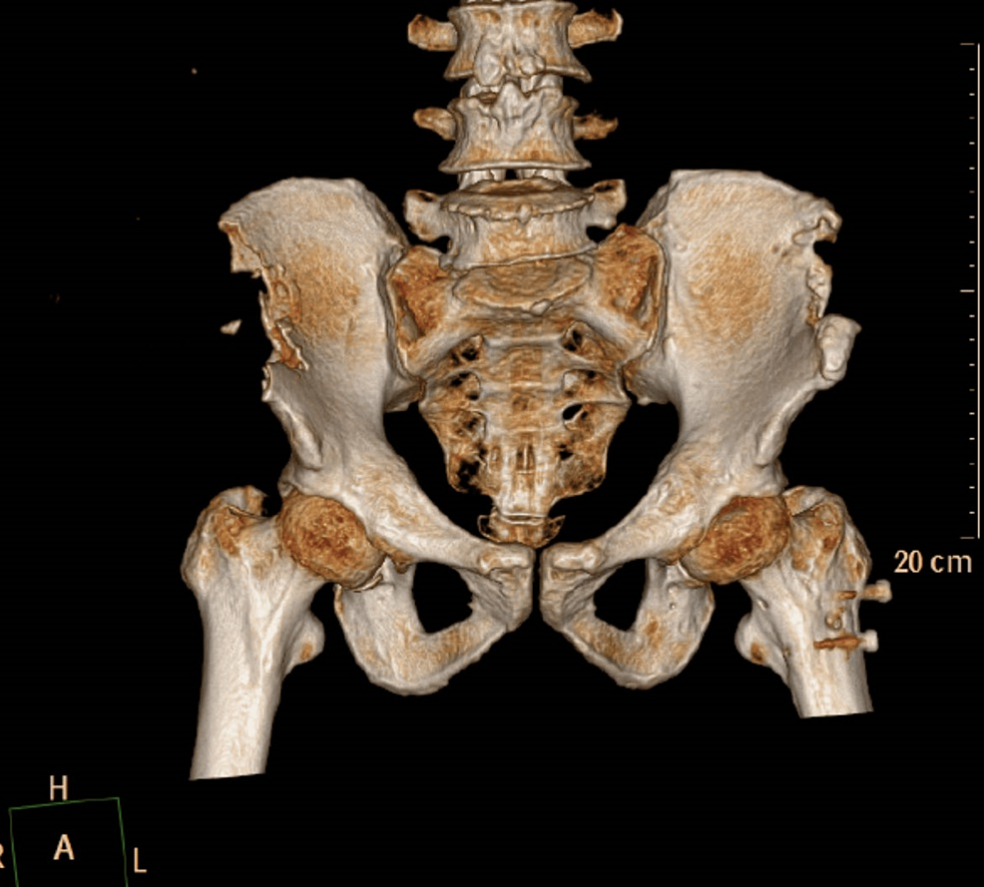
Three-dimensional CT reconstruction showing the postoperative state of bilateral iliac crest bone harvesting and the residual part of the right iliac crest was sharp
